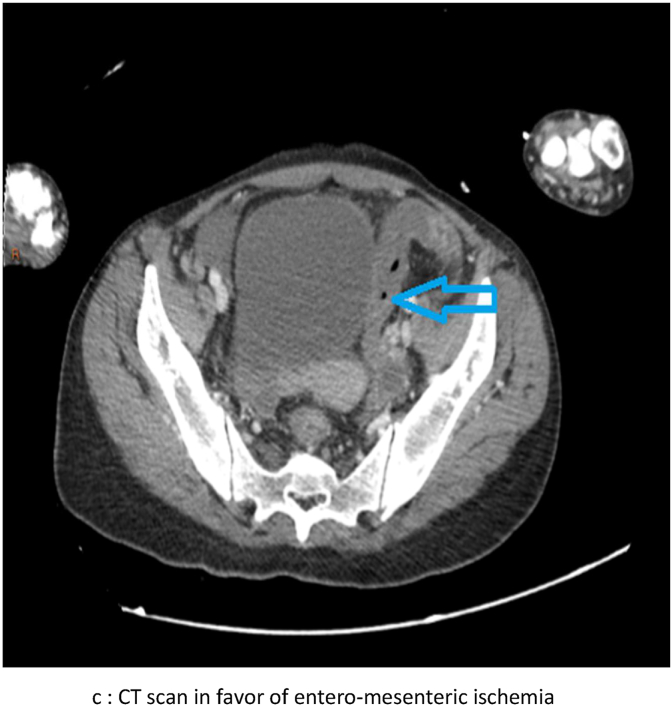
CT scan in favor of entero-mesenteric ischemia.
